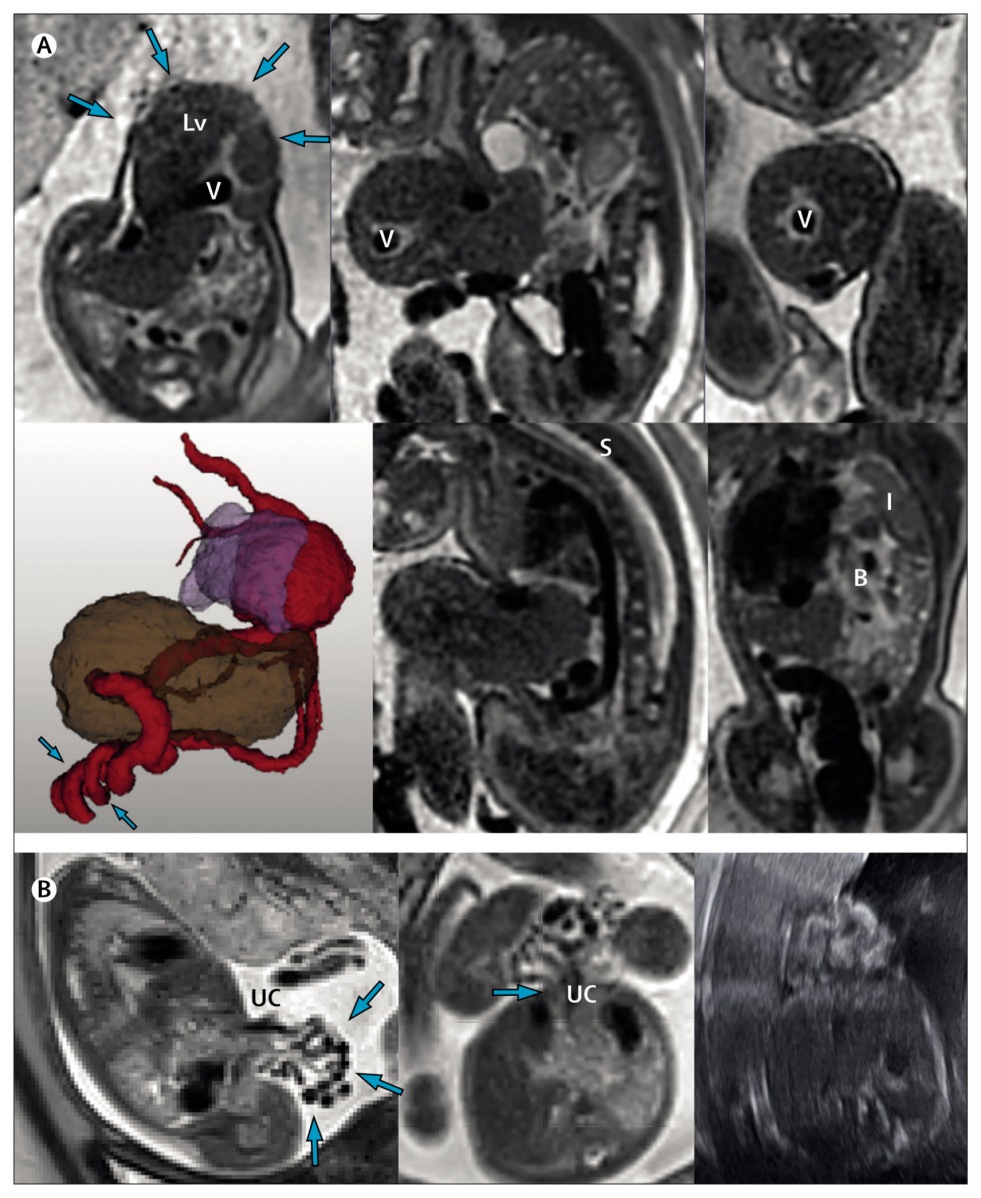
MRIs of two fetuses with abdominal wall defects (A) Fetus at 34 weeks and 6 days of gestation with exomphalos (arrows on axial image, top left). The 3D image shows that the exomphalos contains the liver (brown) and the prominent umbilical vein (red) follows a twisting and irregular course (arrows; lung shown in lilac). The lower middle panel shows marked angulation of the thoracic spine. Bowel in the associated diaphragmatic hernia is shown in the lower right image with the compressed left lung. (B) Gastroschisis, with no sign of bowel dilatation on the MRI. The exteriorised bowel (arrows, left image) and insertion (arrow, central image) of umbilical cord are shown. Image on the right shows the referring ultrasound scan. B=bowel. I=lung. Lv=liver. S=spine. UC=umbilical cord. V=vein.
